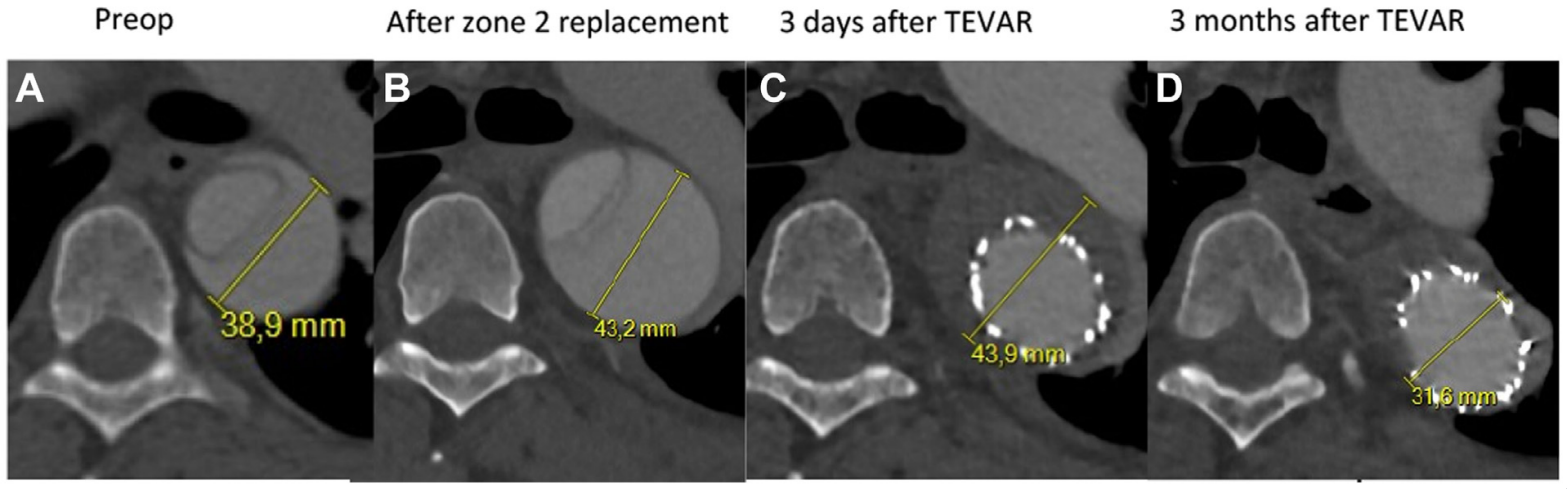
Coronal view of the computed tomography (CT) scans all at the same level (trachea bifurcation) showing dissection with total diameter preoperatively (A) and postdissection dilatation (B), result after thoracic endovascular aortic repair (TEVAR) (C) and favorable remodeling 3 months after TEVAR (D).
